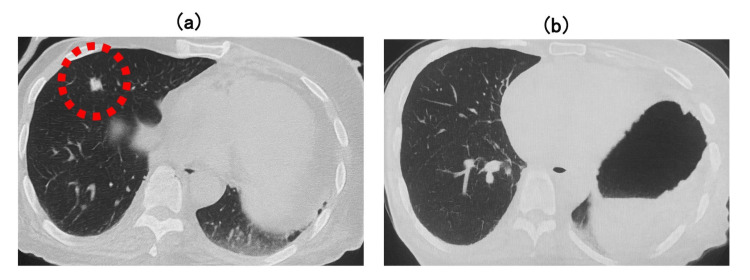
Chest CT images. (a) Computed tomography (CT) before pembrolizumab therapy showing a metastatic pulmonary lesion (red dotted circles). (b) CT scan obtained at the time of the initial syncopal episode showed no evidence of new lesions or progression of pulmonary metastases.
